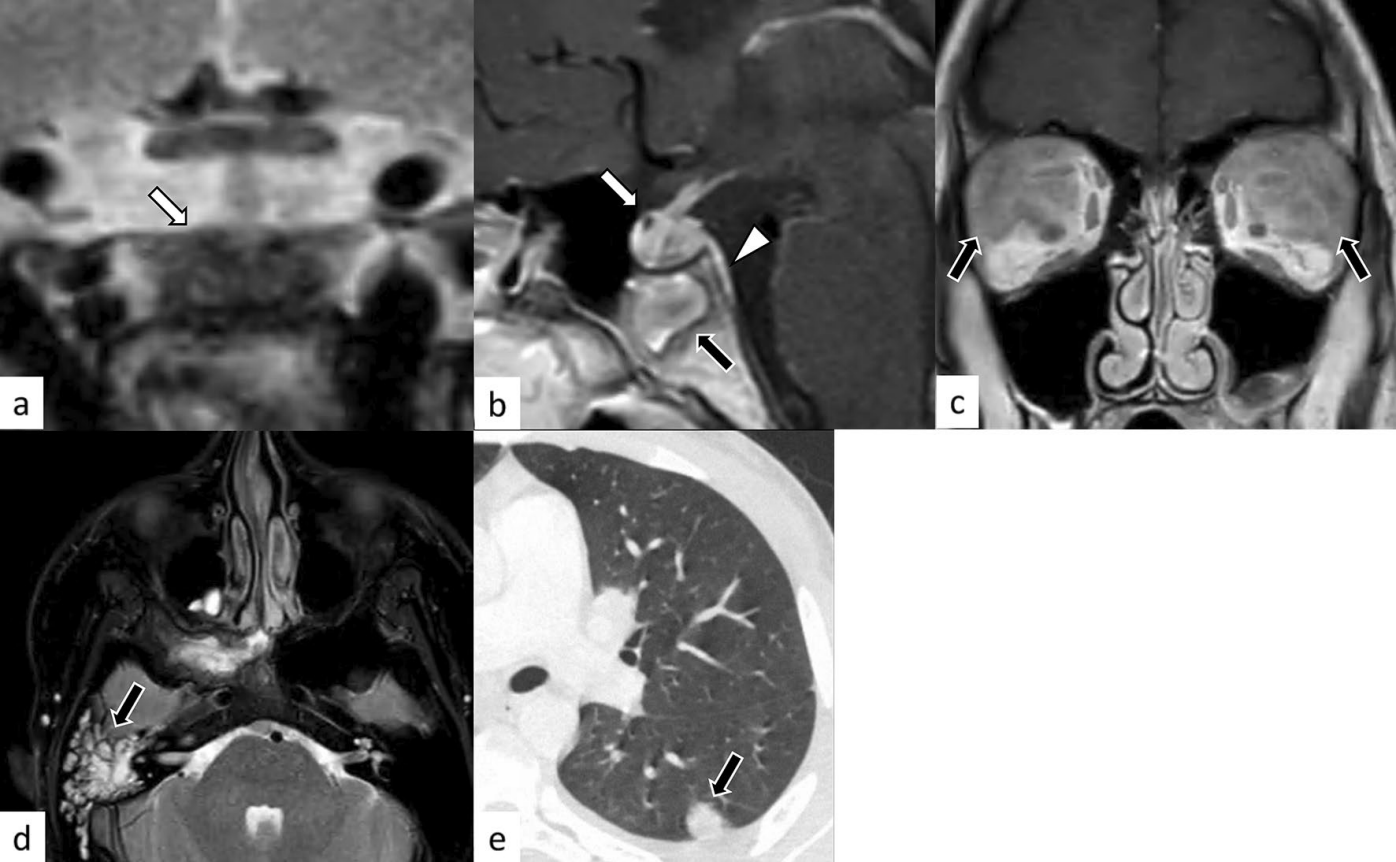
A 20-year-old man with proteinase 3-antineutrophil cytoplasmic antibody-positive GPA. The pituitary gland is enlarged and shows heterogeneously hypointensity on T2-weighted coronal image (a, white arrow) with heterogeneous enhancement (b, white arrow), extending posteriorly along the surface of the clivus (b, white arrowhead). The sphenoid sinus (b, black arrow), bilateral lacrimal glands (c, black arrows), right mastoiditis (d, black arrow), and left lung (e, black arrow) are also involved
